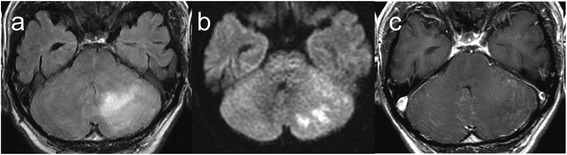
Brain magnetic resonance imaging performed on admission. The findings on diffusion weighted imaging (DWI) mimicked arterial infarction of the posterior inferior cerebellar artery accompanying strong perilesional edema with almost no enhancement of the lesion. a Fluid-attenuated inversion recovery (FLAIR) image on admission. b DWI. c T1-weighted imaging with gadolinium enhancement
